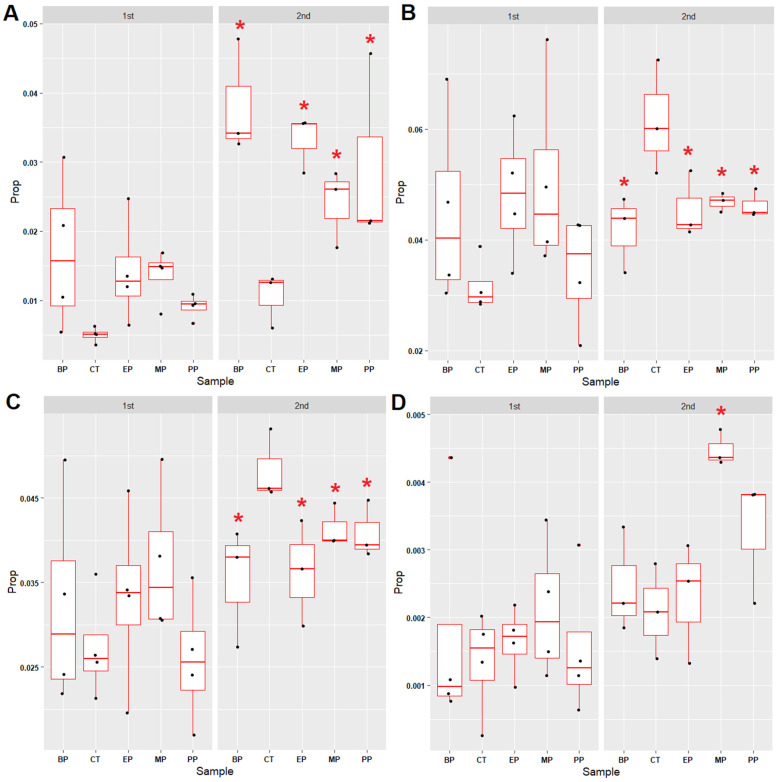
Proportion changes of S cycle-associated microbial communities in the paraben-treated river sediments. (A) Thiosulfate oxidation (M00595: thiosulfate => sulfate). (B) Assimilatory sulfate reduction (M00176: sulfate => H_2_S). (C) Sulfate-sulfur assimilation (M00616). (D) Dissimilatory sulfate reduction (M00596: sulfate => H_2_S). “1st” indicates the meaning of the period between week 0 and week 8. “2nd” indicates the meaning of the period between week 8 and week 17. Red star indicates the *p* value of the Mann–Whitney U test < 0.05 (compared with control (CT)). Prop: proportions of microbial genera. “M00xxx” indicates the meaning of the KEGG module ID number. MP: methylparaben, EP: ethylparaben, PP: propylparaben, BP: butylparaben, CT: control.
